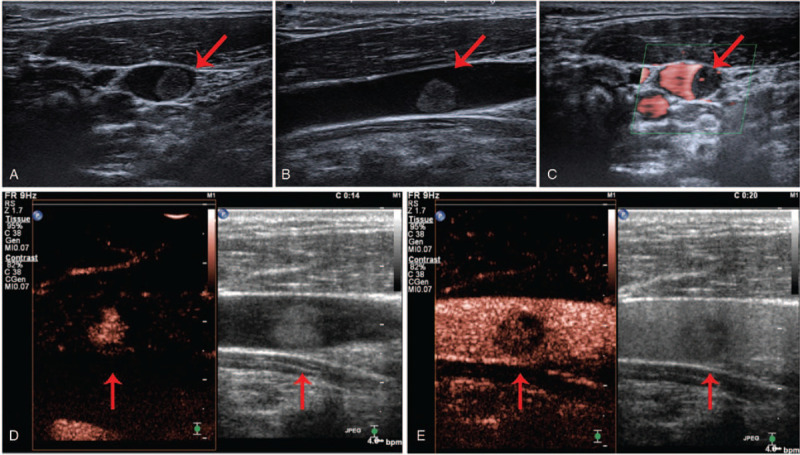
Ultrasonography: The image of the 2-dimensional ultrasound transverse section (A) and longitudinal section (B); (C) The image of the superb microvascular imaging; The images of the arterial phase (D) and the venous phase (E) of contrast-enhanced ultrasonography. The red arrows are pointing to the neoplasm.
